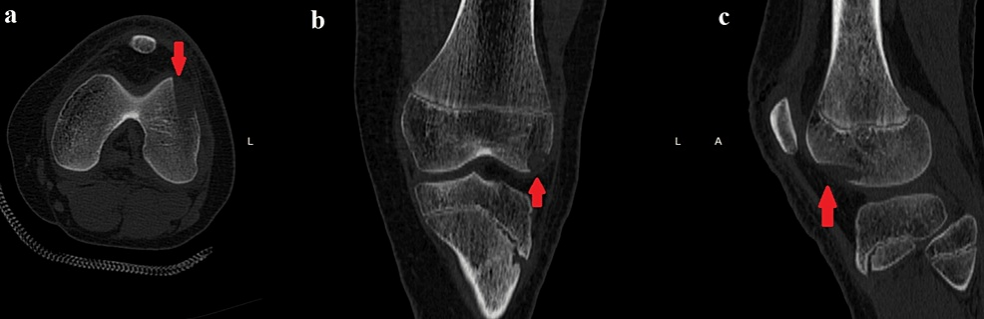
CT scan of the patient’s left knee. The intra-articular lesion is visible on the lateral femoral condyle: (a) axial view; (b) coronal view; and (c) sagittal view. CT, computed tomography
